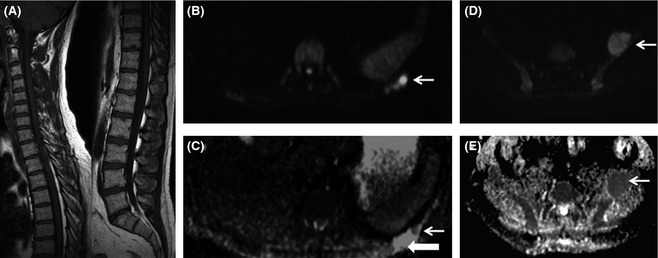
Whole body diffusion weighted magnetic resonance imaging (WB DW‐MRI) can differentiate active from inactive sites and reveals unexpected additional sites of disease. A 53‐year‐old female with a previous history of solitary rib plasmacytoma treated with radiotherapy, with rising paraproteins. Weighted T1 (T1W) MRI of the spine (A) was normal. WB DW‐MRI [axial b900 B, apparent diffusion coefficient (ADC) map, C] demonstrated a 3 mm focal nodule of restricted diffusion (arrows, B and C) within the treated rib bone defect (block arrow, C), in keeping with local recurrence. In addition WB DW‐MRI showed a 5 cm expansile focus of disease arising from the left iliac bone [arrows on b900 (D) and ADC map (E)].
